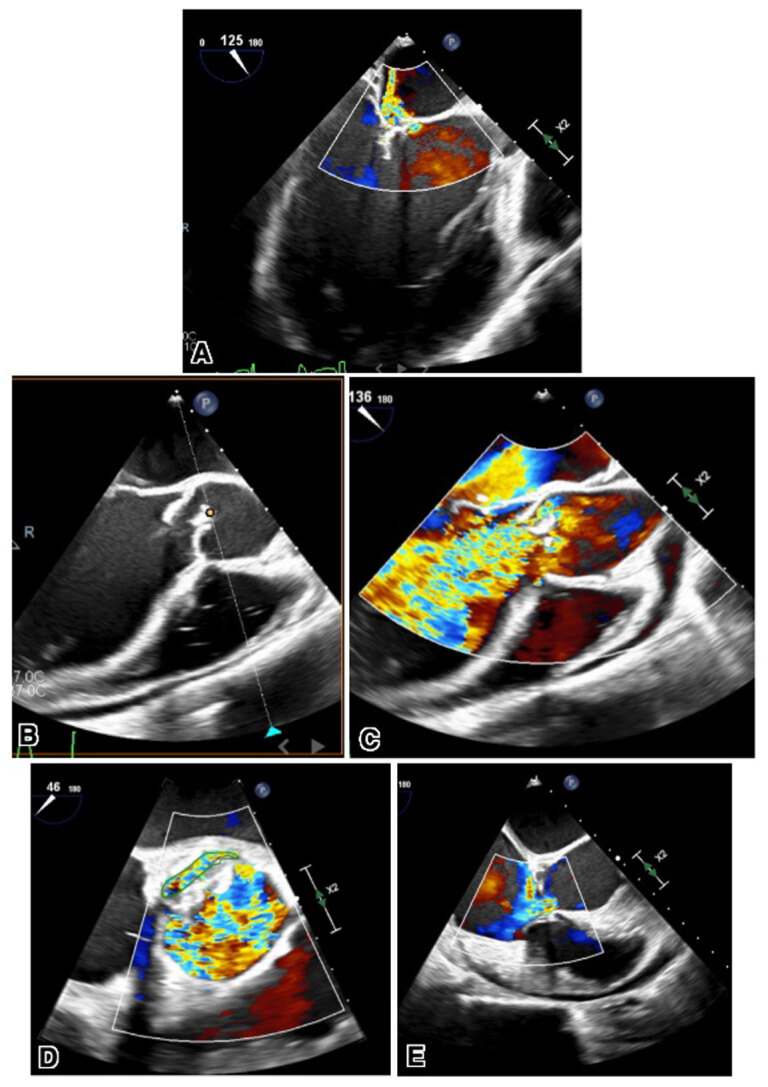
(A) Transesophageal echocardiogram images showing perforated anterior leaflet of the mitral valve. (B) Long- and short-axis views showing perforated aortic valve (C, D) with color flow Doppler. (E) Transesophageal echocardiography long-axis view with color flow Doppler showing ventriculoatrial shunt flow from the left ventricle into the right atrium.
